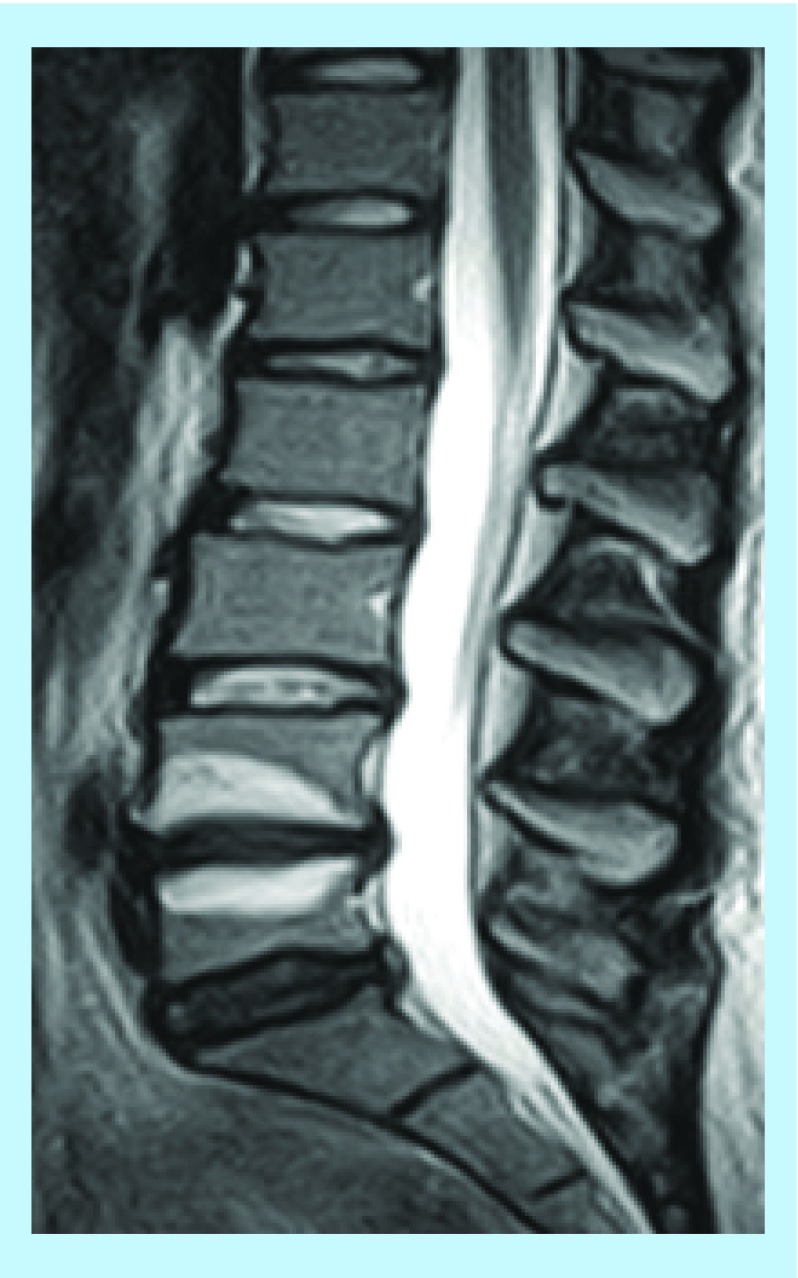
An MRI T2-weighted image. Typical Modic changes type 1 (high signal intensity) in a lumbar segment. Reproduced with permission from [4].
